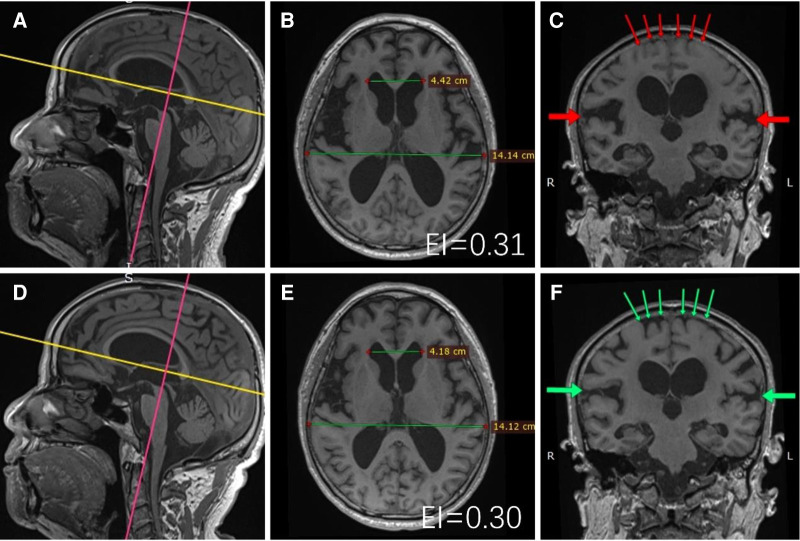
A 72-year-old male patient underwent T1-weighted thin-layer head MRI scans before and after surgery. Multiplanar reconstructions of the data are shown above. Preoperative: (B,C) were reconstructed along the yellow and pink lines of (A), respectively; Postoperative: (E,F) were reconstructed along the yellow and pink lines of (D), respectively. EI measurements (EI is the ratio of the maximal distance of frontal horns to the maximal inner diameter of the skull on the same plane) were performed at the same transversal planes, the postoperative (B) EI decreased compared with that of preoperative (E); Signs of DESH were observed in the same coronal planes, the degree of postoperative convex subarachnoid space tightness (green thin arrows of F) was reduced compared with that of preoperative (red thin arrows of C), and the degree of postoperative Sylvian fissure enlargement [green thick arrows of (F)] was reduced compared with that of preoperative [red thick arrows of (C)]. The patient's preoperative mRS was level 4, which improved to level 1 one year after LPS surgery.
